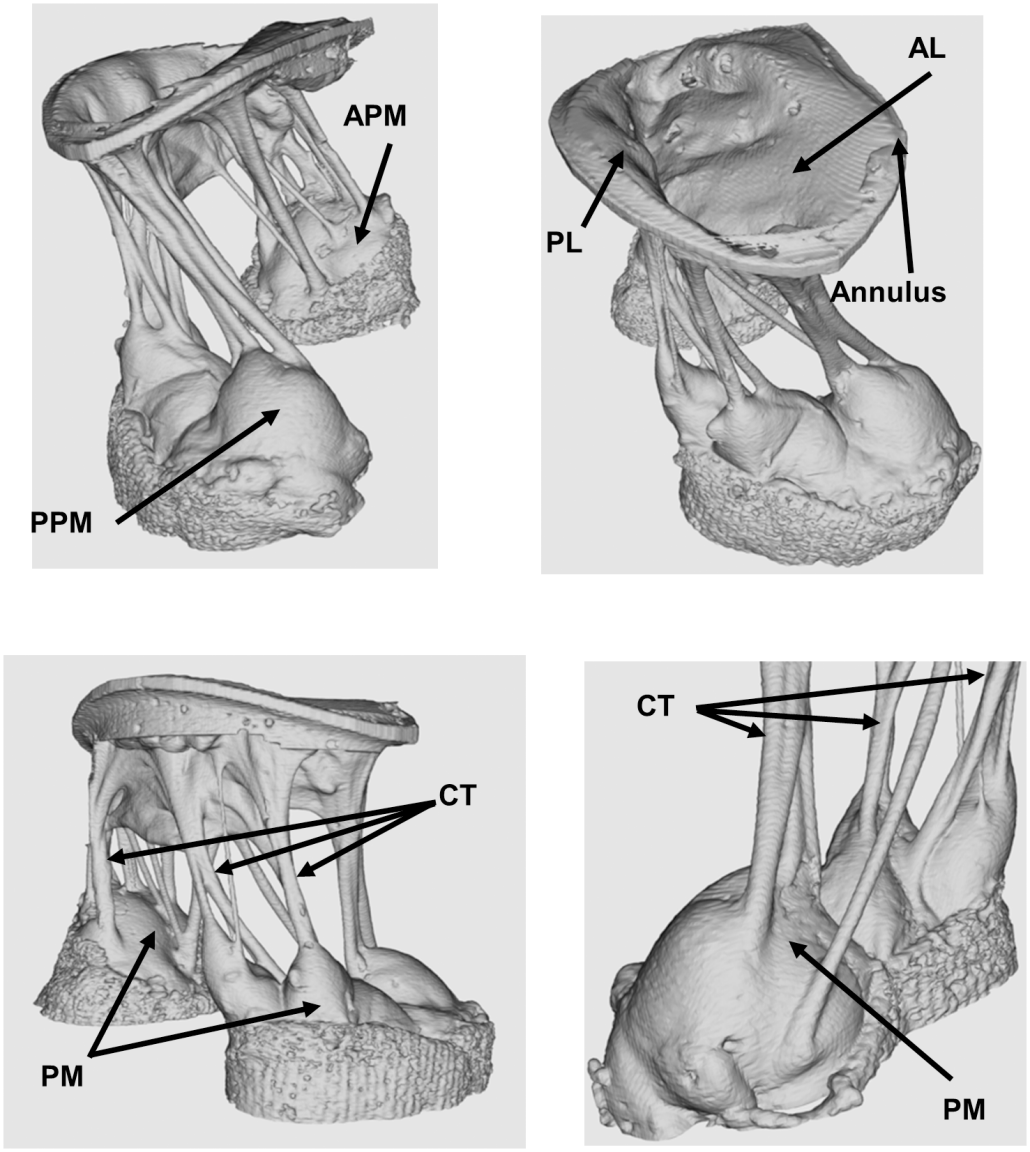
Example of the high level of details of the mitral valve structures obtainable by 7T MRI imaging. Ventricular and atrial views displayed, as well as enlarged views of the papillary muscles and chordae tendineae. The valve is 100% slack in a zero-pressure state. APM: Anterior Papillary Muscle, PPM: Posterior Papillary Muscle, CT: Chordae Tendineae, PM: Papillary Muscle, AL: Anterior Leaflet, PL: Posterior Leaflet.
